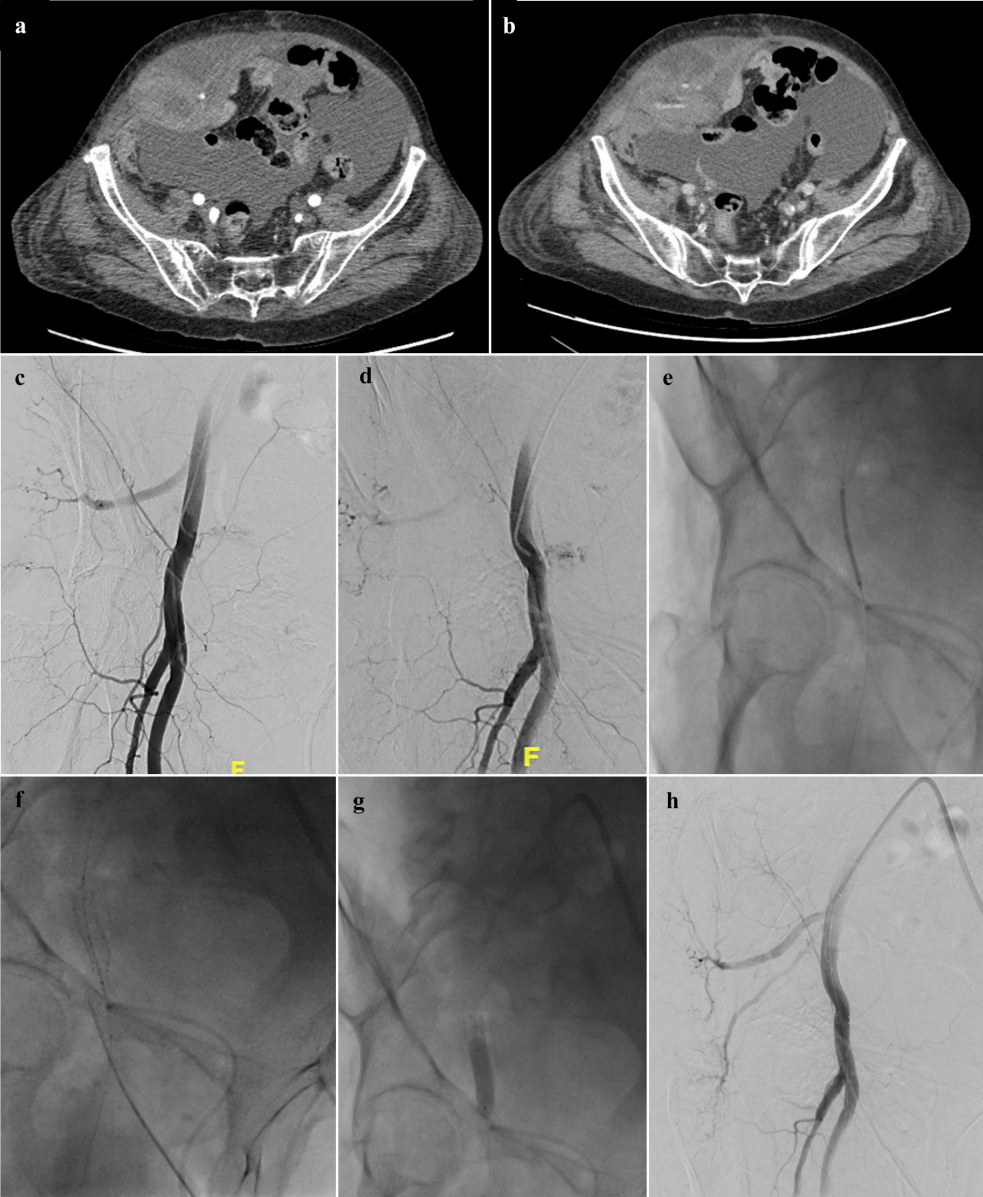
CT and DSA images of a technically failed PTE of the target vessel of a spontaneous AWH. Clinical success was anyway reached by a covered stent placement. Axial CT images after contrast-enhanced acquisition at arterial and late phase showed a large hematoma within the right rectus abdominis with active arterial bleeding (a–b). At DSA, active contrast extravasations from small multiple proximal branches of right inferior epigastric artery were detected (c–d). Unable catheterization, due to extensive ostial calcifications of right inferior epigastric artery and 8 mm covered stent placement at distal segment of right iliac external artery (e). Stent deployment and balloon post-dilation (f–g). At final DSA, no signs of active bleeding were detected (h)
